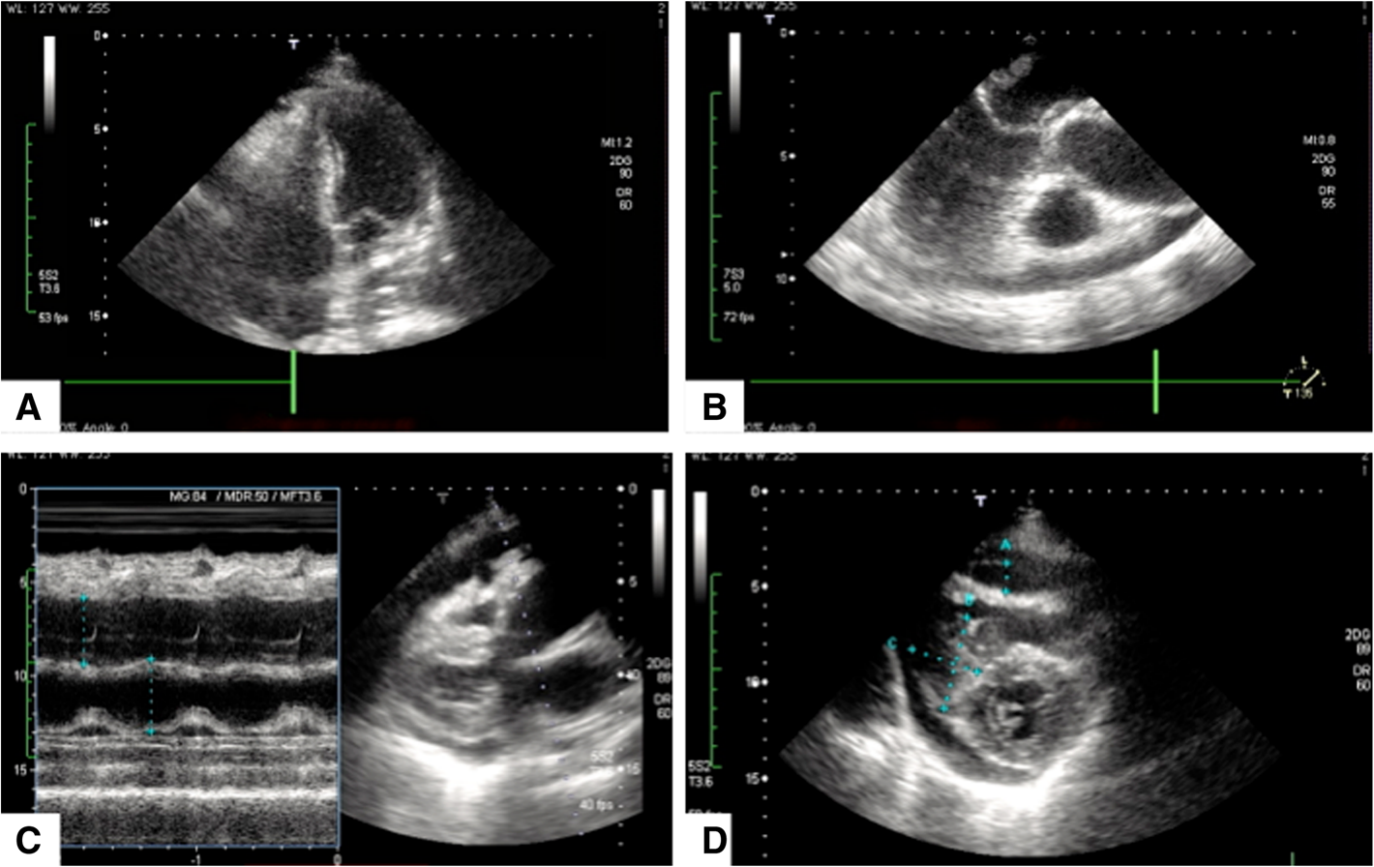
Initial transthoracic echocardiography (TTE) study showing no structural abnormalities on all views. A 4-chamber view. Regular ventricles and atria, no suspicious masses can be identified. Intact mitral and tricuspid valvular apparatus. B Parasternal long axis showing a regular, non obstructed left ventricular outflow tract. Left Atrium, mitral valve with anterior and posterior leaflet, left ventricle, aortic valve and ascending aorta. No ventricular wall dyskinesias, no obstructions. C Aortic root and left atrial diameter measurement. 35,5 mm versus 38.5 mm D Parasternal short axis. Mitral valve level showing intact valvular apparatus, no vegetations can be identified.
